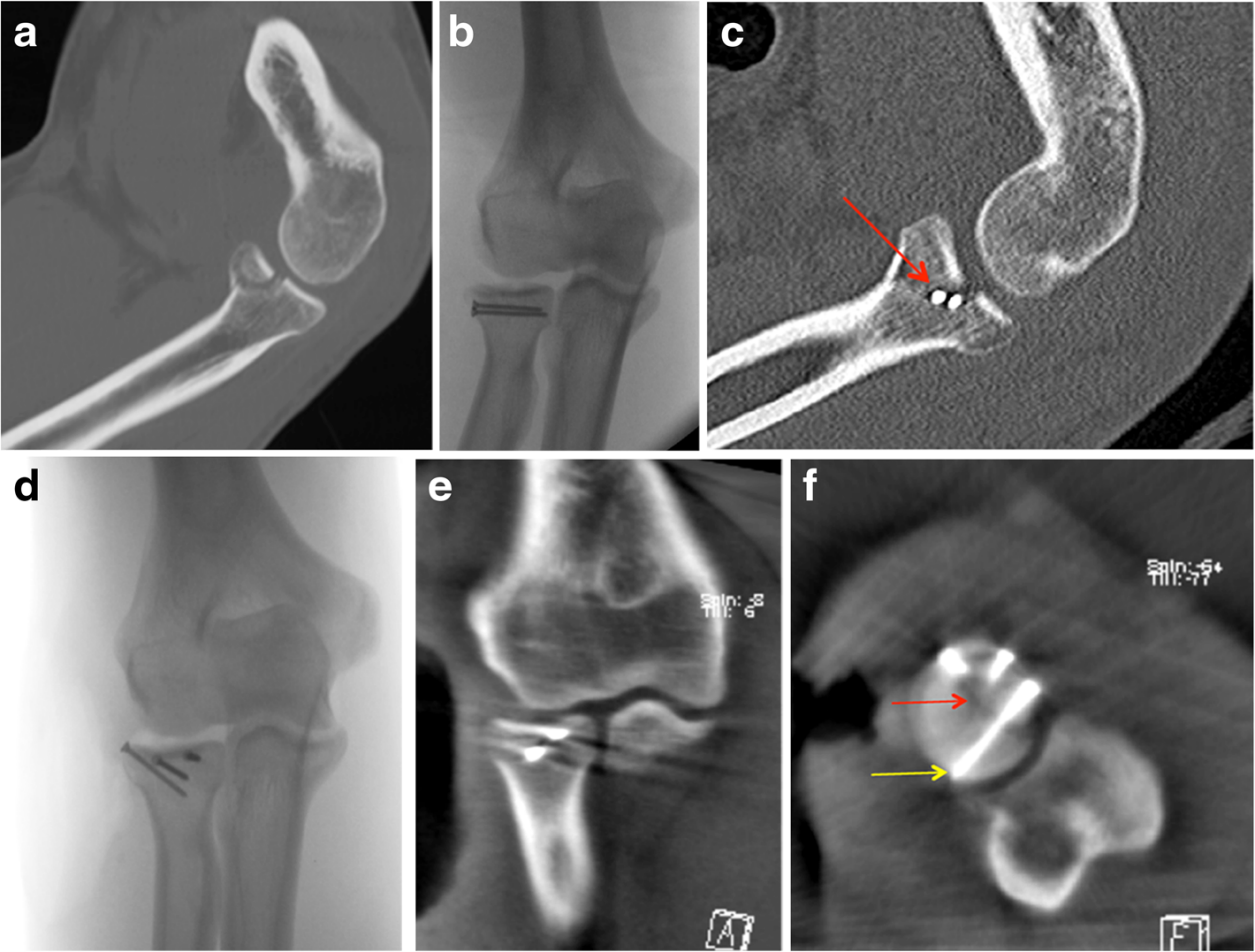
a Pre-operative computed tomography showing a radial head fracture type II (Mason). b Intraoperative 2D fluoroscopy images after open reduction and fracture fixation with screws showing anatomic reduction. c Postoperative computed tomography revealed a remaining step in the joint surface. Both screws were placed into the fracture line, which was not seen on conventional 2D fluoroscopy. d During an index operation, the screws were replaced after open reduction. e Intraoperative 3D imaging confirmed the anatomical reduction and correct screw positioning in the coronal multi-planar-reconstructions. f Intraoperative 3D imaging confirmed the anatomical fracture reduction (*red arrow*) and correct screw positioning und length (*yellow arrow*) in the axial multi-planar-reconstructions
